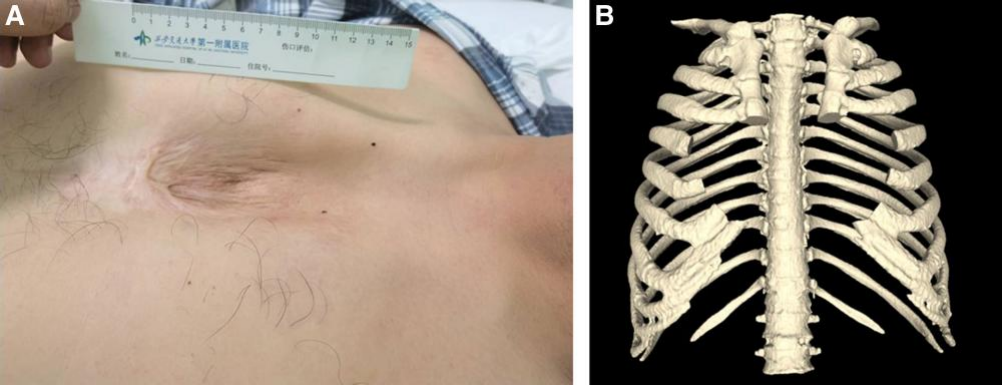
On examination, the midline of the anterior chest wall presented as a sunken V-shaped area with thin skin (*A*). Chest computed tomography 3D reconstruction confirmed a high cardiothoracic ratio and a complete sternal cleft (*B*).
